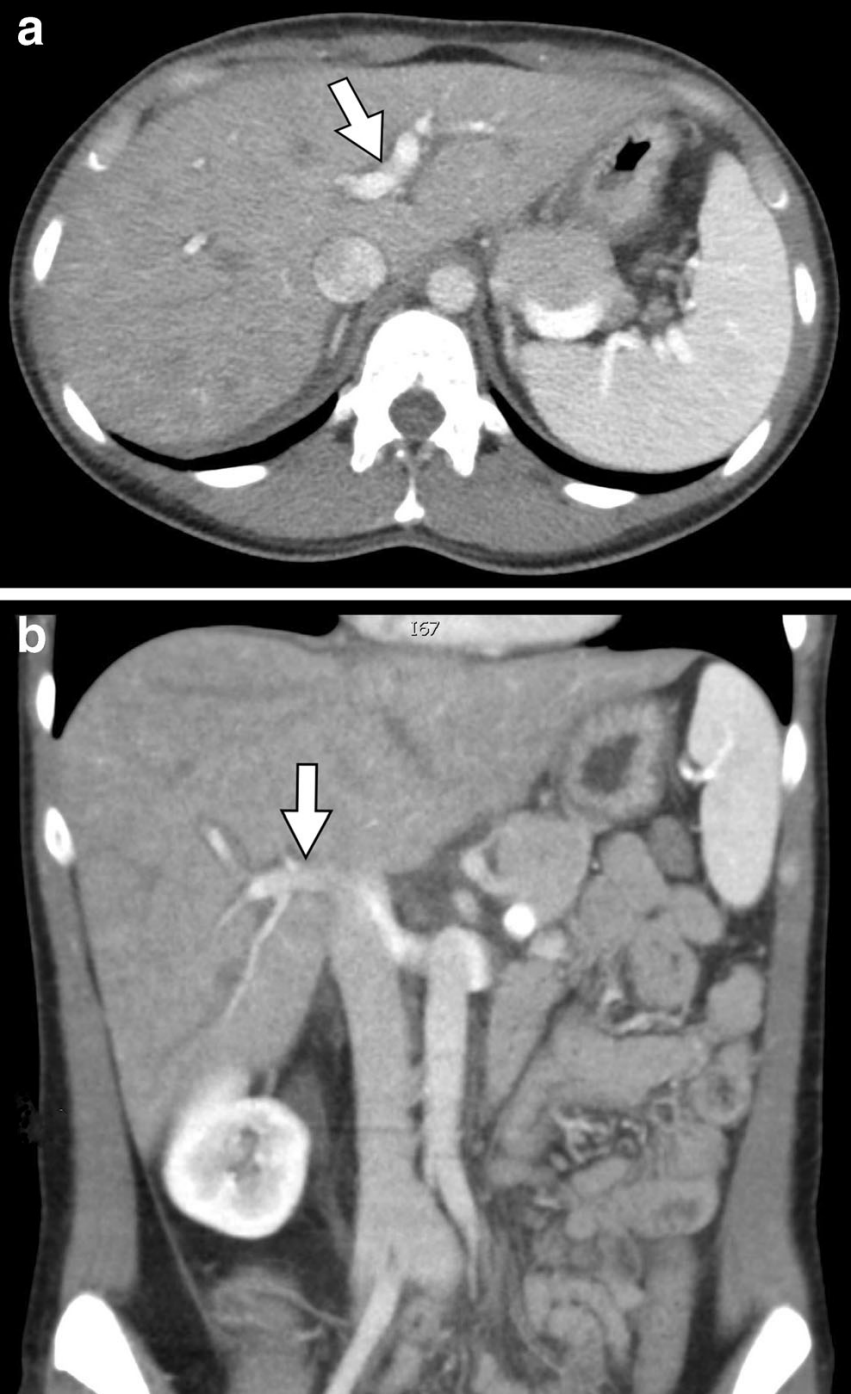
A 54-year-old male with acute myelogenous leukaemia treated with alemtuzumab. a, b Axial and coronal contrast-enhanced CT images, respectively, demonstrating hepatomegaly and periportal oedema (*arrows*) indicative of acute hepatitis
